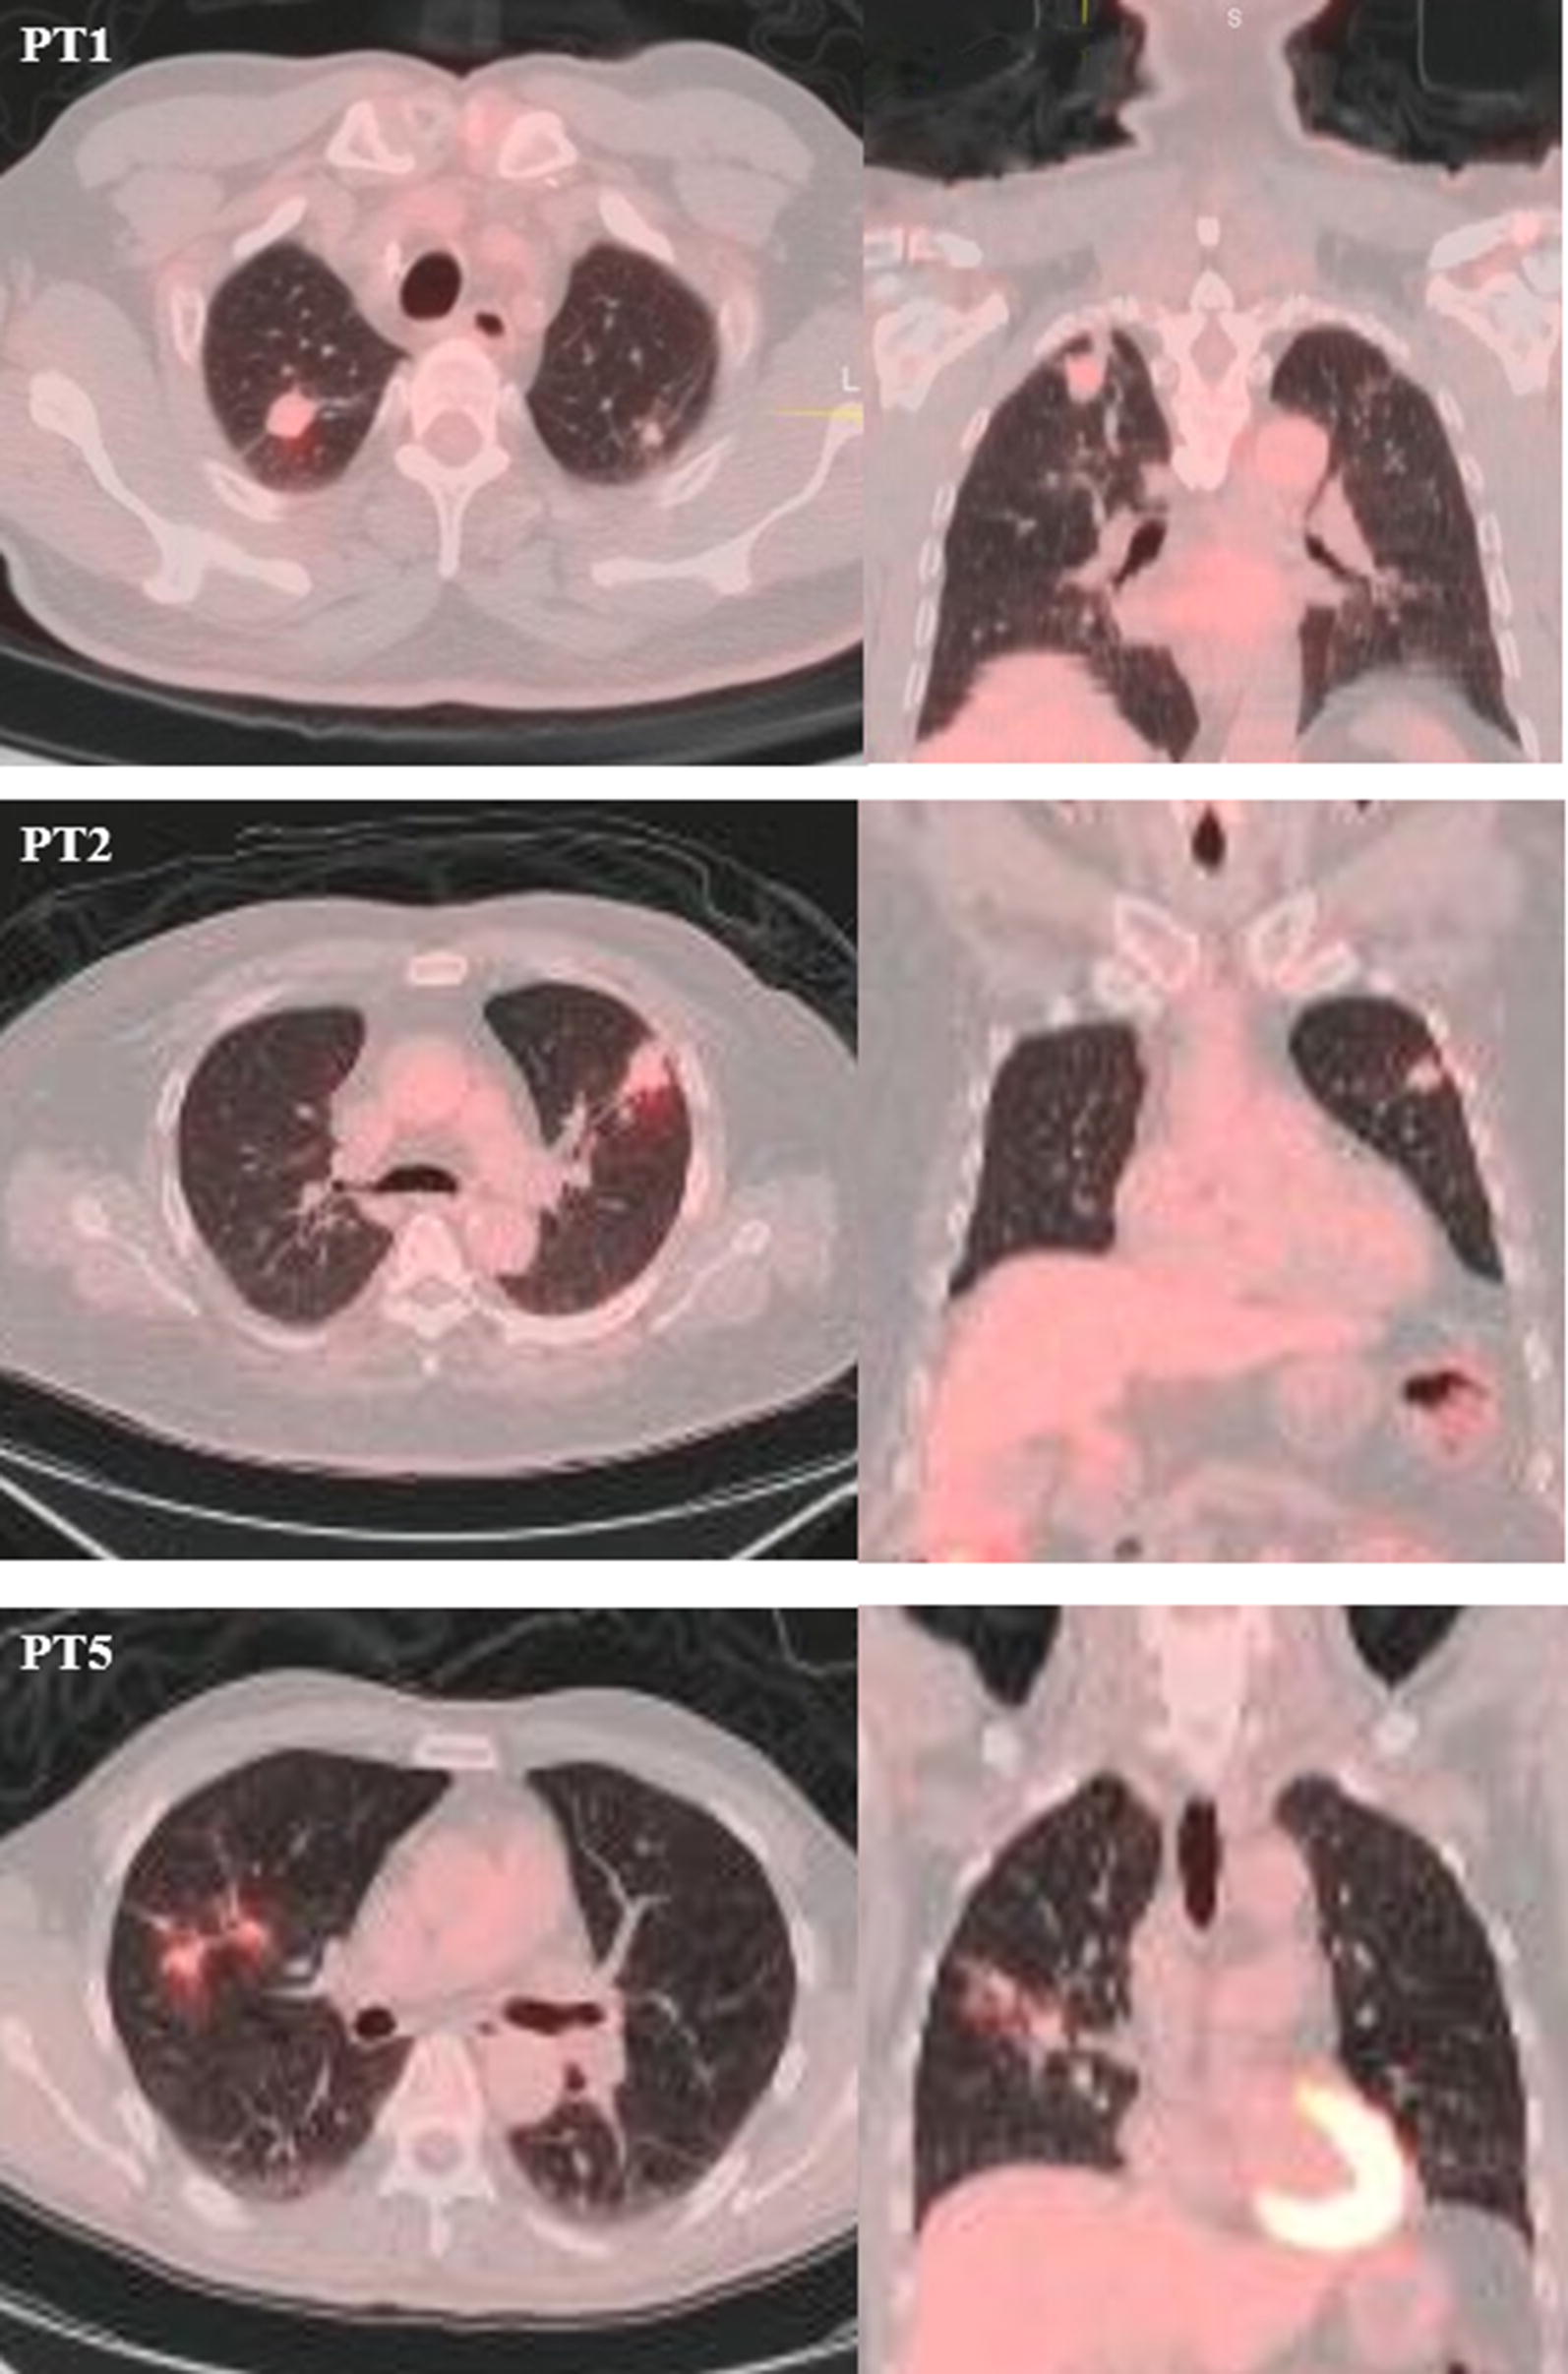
FDG-PET/CT scans of patients (PT) 1, 2, and 5. Images are axial and coronal fused PET/CT images with CT in standard lung windows and the PET in an orange color scale. PT1 shows a 2 cm spiculated lung nodule in the right upper lobe (RUL) with SUV_max_ of 2.72. PT2 shows a similar finding in the left upper lobe (LUL) but tracer uptake is slightly higher with SUV_max_ of 3.9. PT5 shows two RUL nodules, with 1.6 and 1.4 cm, both with SUV_max_ of 2.6
